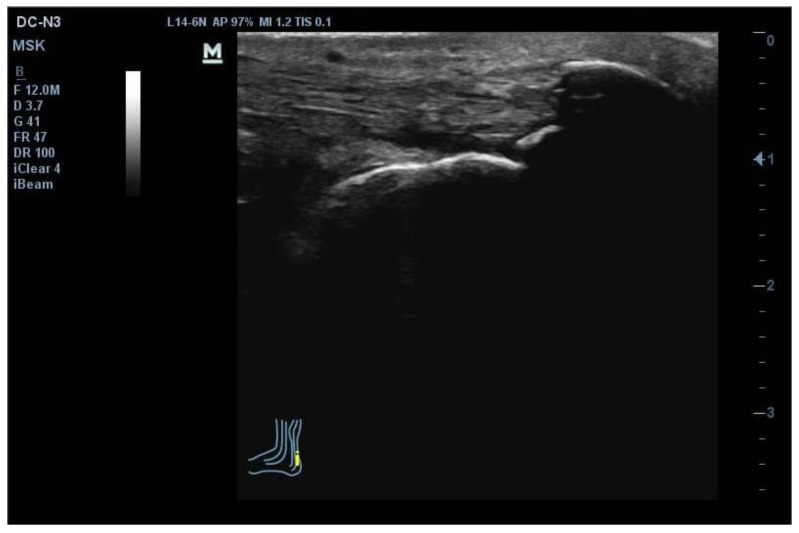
Achilles complex ultrasound assessment image. The blue and yellow lines are the anatomical structure and the ultrasonographic linear transducer position.
